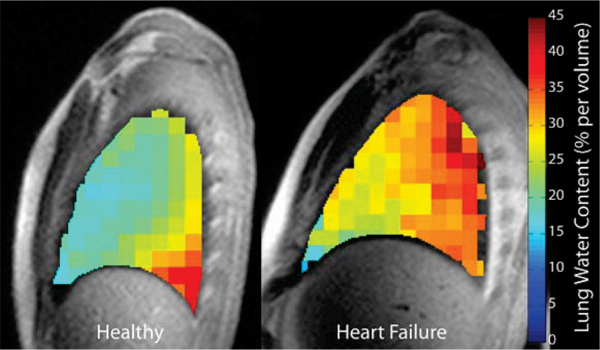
MRI-derived lung water (mid-sagittal right lung) for a healthy subject (total average lung water 21.7%) and a heart failure patient (37.2%).
